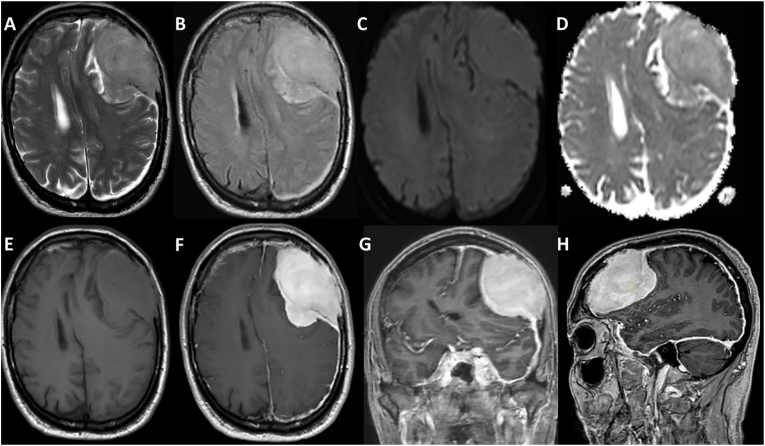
Magnetic Resonance Imaging (MRI) at presentation Axial T2-weighted (T2-w) (A), FLAIR (B), DWI (C), ADC (D), non-contrast axial T1-weighted (T1-w) (E) and axial, sagittal and coronal post-gadolinium T1-w images (F, G, H) are shown. A large extra-axial mass is shown with intermediate signal intensity on T2-w. No diffusion restriction is present. Only mild edema is present. There is significant mass effect with subfalcine herniation. There is osseous and focal dural involvement, with striking thickened and enhancing dura along the left hemispheric convexity. Note that the dura is located within the tumor and thus detached from the skull by the tumor.
